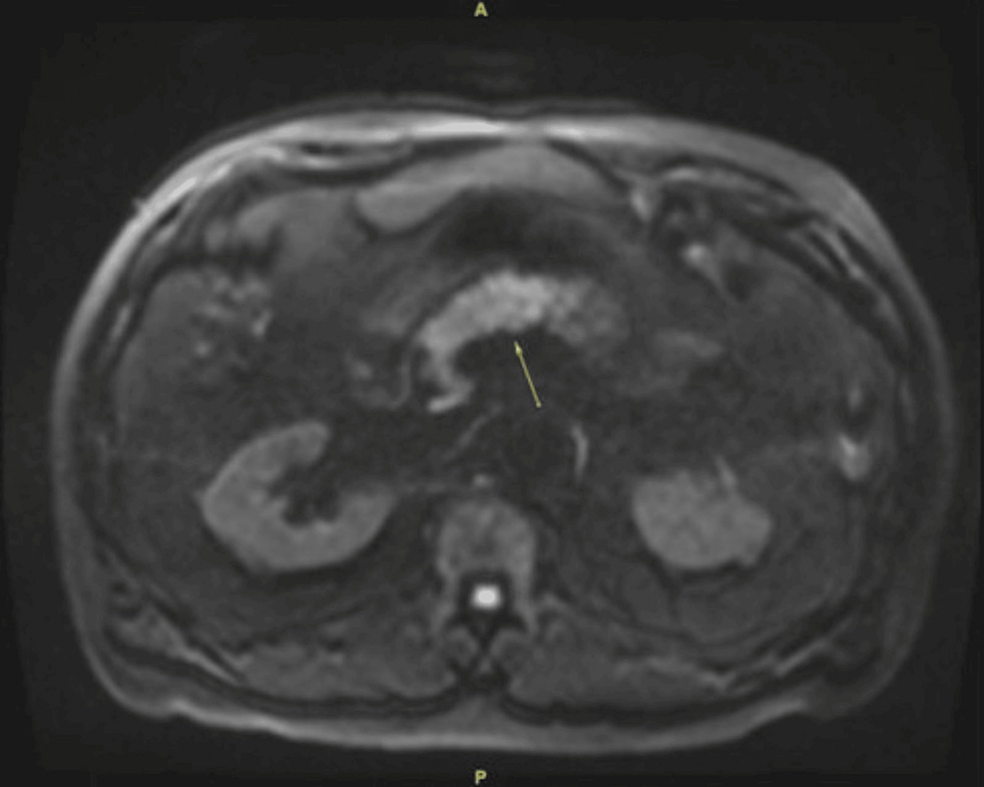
MRCP image from the second episode of pancreatitis Figure 2 shows an MRCP image from the second episode of pancreatitis. There is again a mildly increased DWI signal in the pancreatic head and body in Figure 2 (arrow), which signifies acute pancreatitis. MRCP: Magnetic resonance cholangiopancreatography; DWI: Diffusion-weighted imaging
